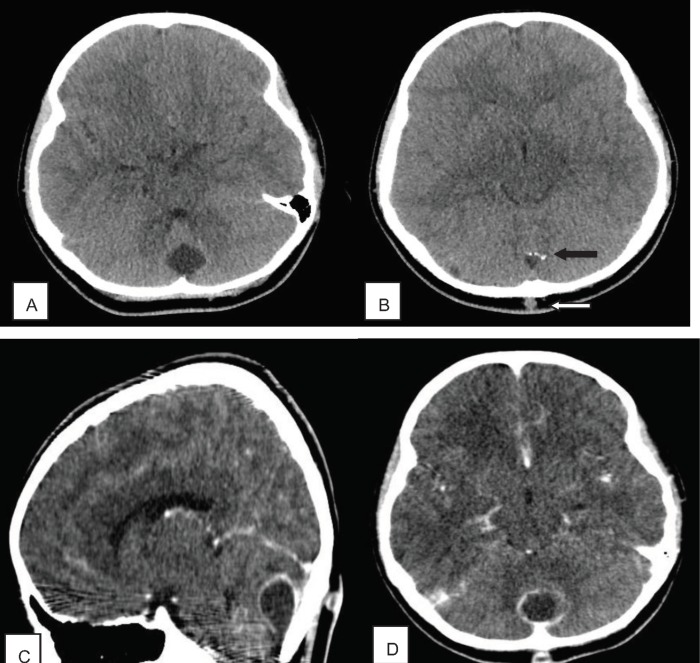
A and B axial non-enhanced brain CT scan show an extra-axial hypodense relatively round lesion in posterior mid aspect of posterior fossa. Note the calcified foci in superior aspect of the lesion(black arrow) as well as a small subcutaneous lesion in the overlying scalp(white arrow)
